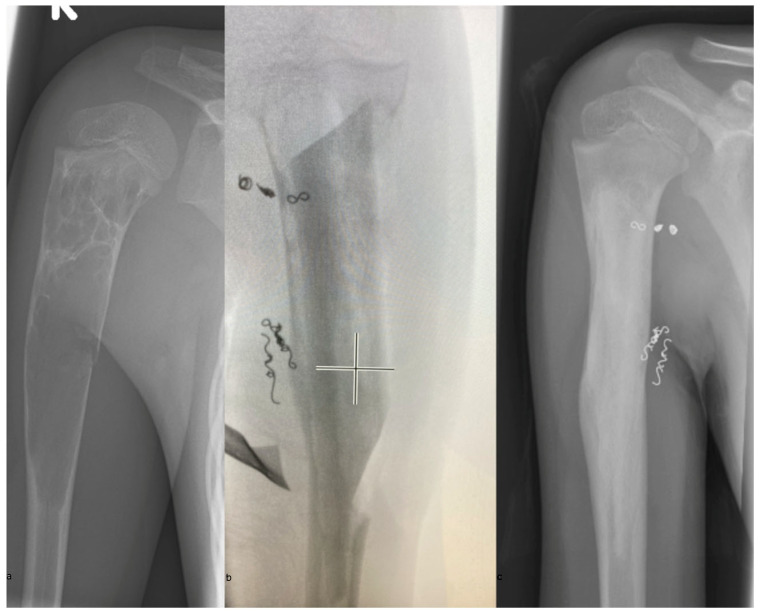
Preoperative X-ray, showing the expansion of the cyst and pathological fracture (a), intraoperative fluoroscopy after reconstruction (b) and complete consolidation of the humerus one year postoperatively (c).
